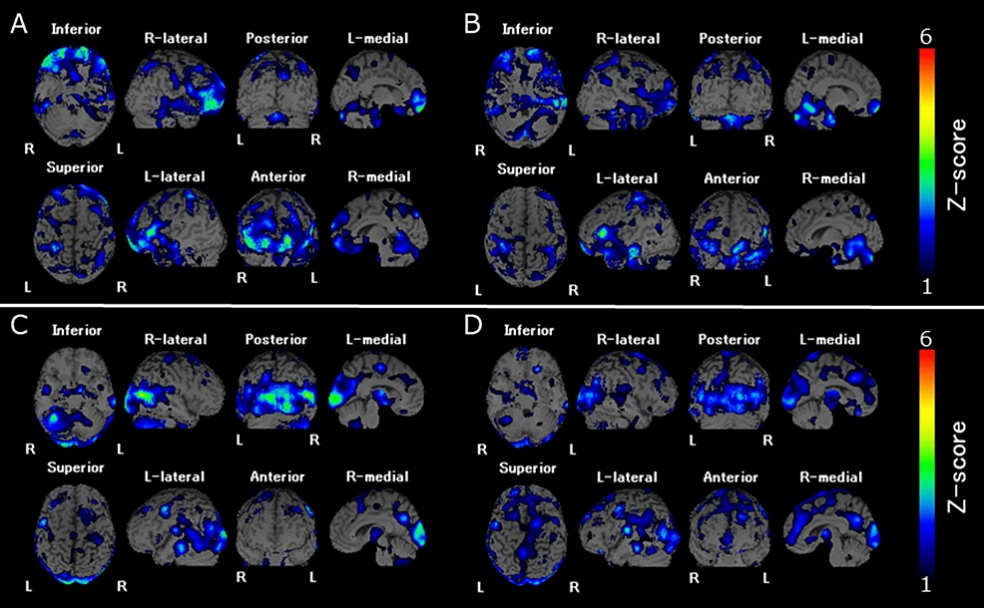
Increase in rCBF with (A) and without Cotard’s syndrome (B), and decrease in rCBF with (C) and without Cotard’s syndrome (D). rCBF: regional cerebral blood flow; SPECT: single-photon emission computed tomography.
